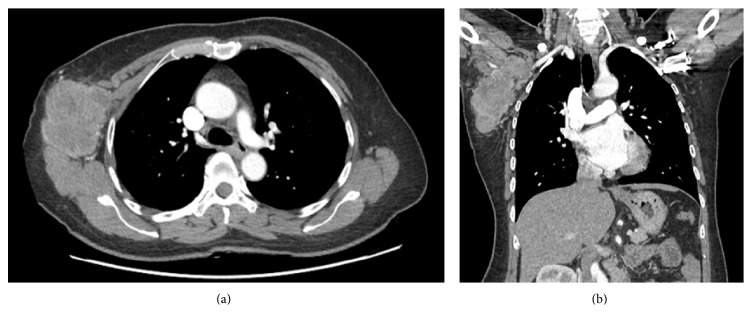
Axial (a) and coronal (b) chest computed tomography images show a 12 cm mass with a lobulated contour and heterogeneous enhancement in the right axilla.
